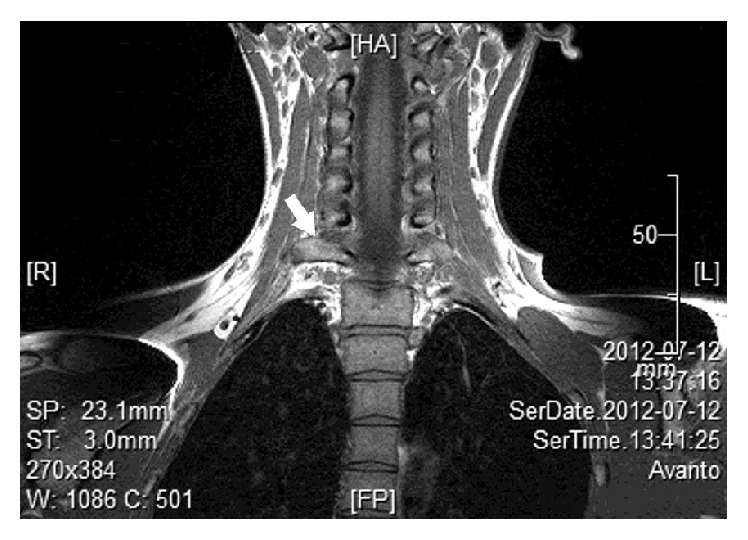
A magnetic resonance imaging (MRI) coronal scan demonstrating unilateral cervical rib (arrow).
